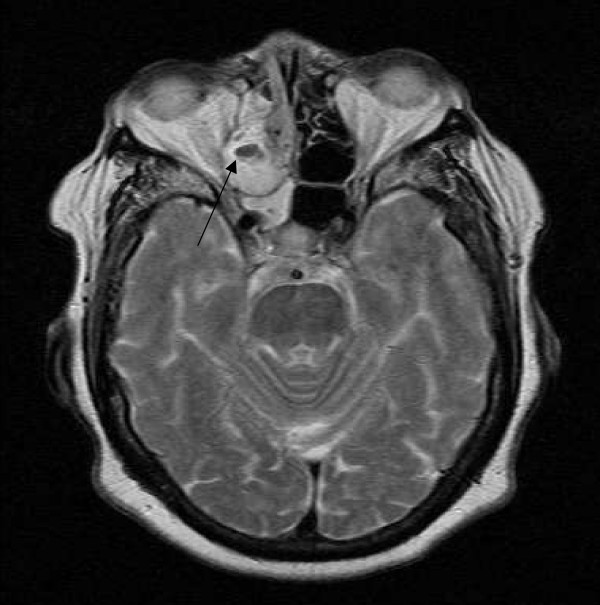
MRI revealed a large polypoid tumor mass eroding through the cribriform plate of the right ethmoid sinus into the cranial cavity.
